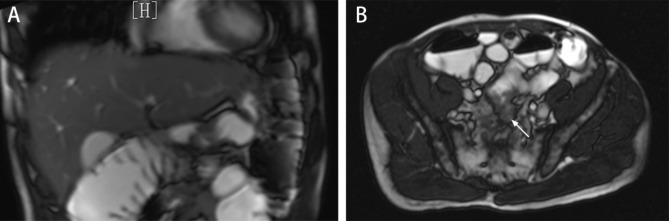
Preoperative imaging data A: MRI showed no metastatic lesions in the liver. B: MRI showed that the lesion was located in the lower part of the rectum(white arrow), and there was no lateral lymph node metastasis in the pelvis
